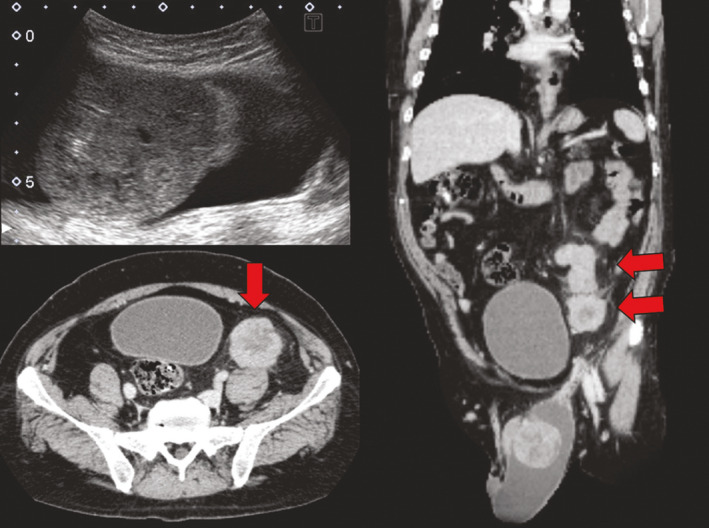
Ultrasonography showed testicular enlargement with a mosaic appearance inside the hydrocele of the testis, which was suspicious for a testicular tumor. CT showed a testicular tumor with a maximum diameter of 6.2 cm inside the hydrocele of the testis. Metastases were found in the pelvic and pararenal aorta lymph nodes, with a maximum diameter of 6.1 cm.
